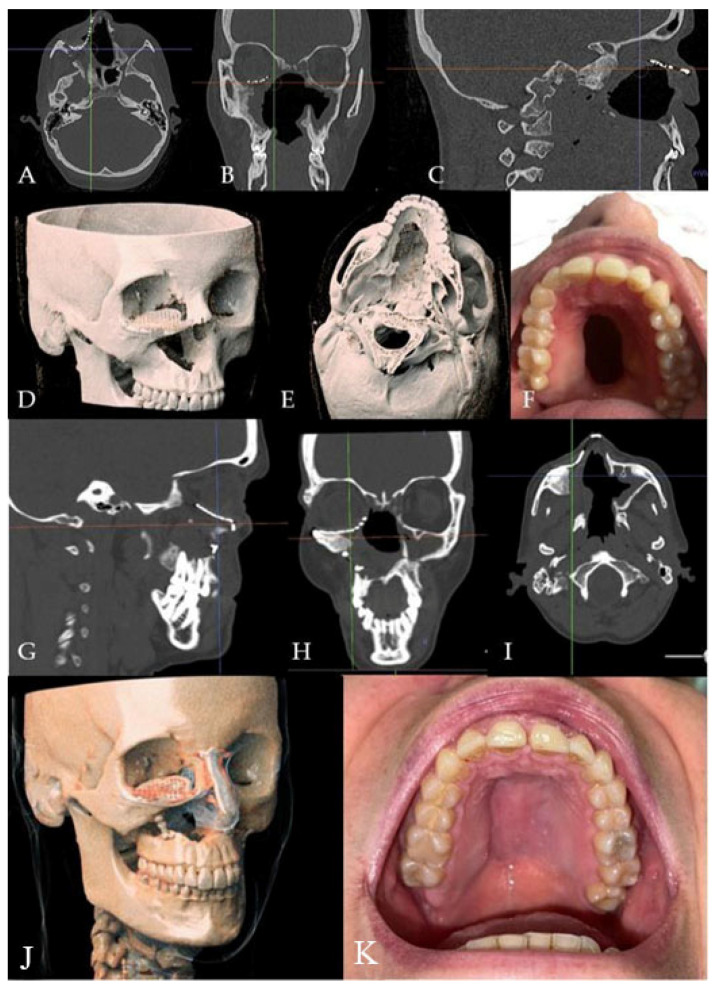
(A–E) Radiographic and 3D reconstruction using InVivo6^®^ and Anatomage Table EDU vers.8^®^ (Santa Clara, CA, USA) software. DICOM files were imported in InVivo^®^ (California, USA) software to obtain the axial, cross, and panorex sections. Afterwards, the DICOM files were imported into Anatomage Table^®^ (Santa Clara, CA, USA) to obtain the 3D rendering. (F) Clinical presentation of the oro-nasal communication. (G–J) Radiographic and 3D reconstructions using InVivo6^®^ and Anatomage Table EDU vers.8^®^ (Santa Clara, CA, USA) software at the one-year follow-up. (K) One-year clinical follow-up.
